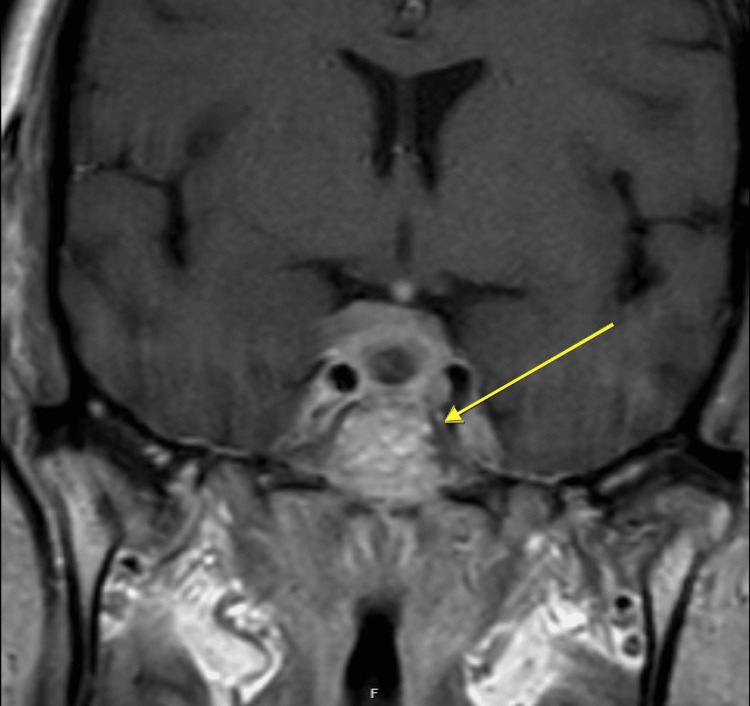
Coronal T1 MRI of the orbits with intravenous gadolinium contrast and fat suppression series demonstrating residual tumor above the sella turcica with invasion into the right cavernous sinus
